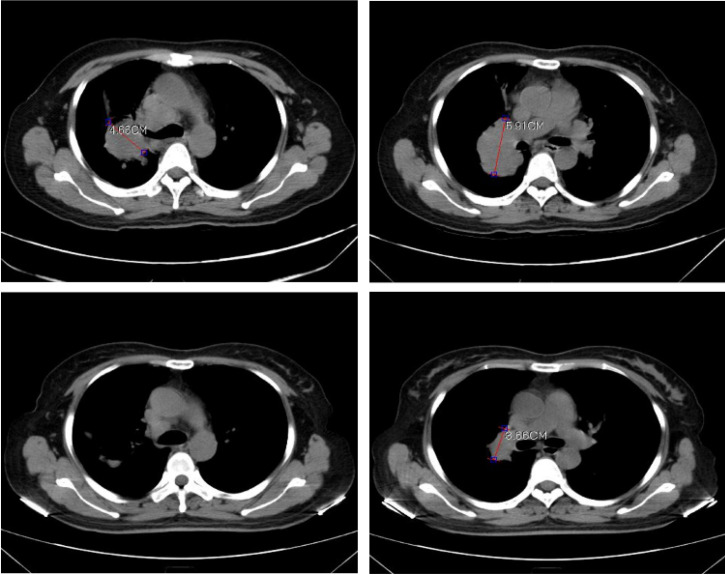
Computerized tomography (CT) scans showing tumor regression in the right upper lobe lesion and the right hilar lesion following treatment with osimeritinib in an extensive-stage SCLC patient harboring *EGFR* L858R and T790M mutations.
